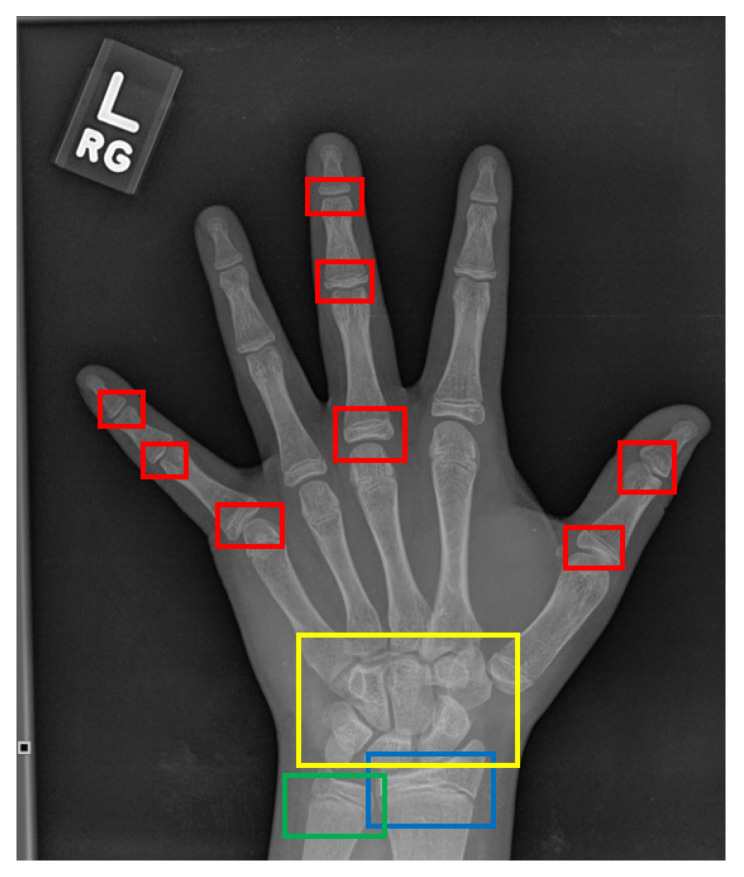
The ROIs of a hand X-ray image for the TW approach. The red boxes refer to the phalangeal joints, the yellow box refers to the carpal region, the blue box refers to the radius physis region, and the green box refers to the ulna physis region.
